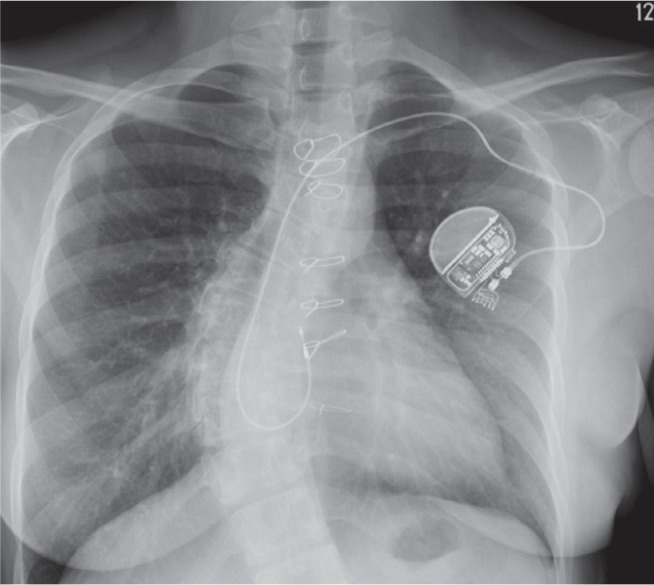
Frontal chest radiograph of a patient with a single-chamber atrial pacemaker.
